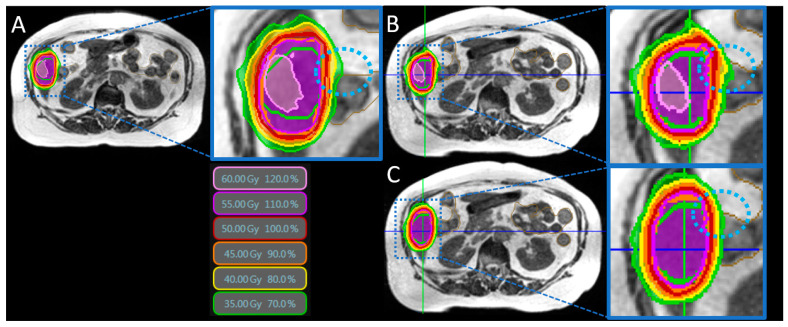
Case study. (A) Baseline plan (50 Gy in 10 fractions) with already slightly impaired PTV coverage (91%) due to proximity to the intestines (brown). (B) Daily online MRI-guidance revealed that the small bowel had moved slightly forward in ventral direction (dashed light-blue circle) leading to a predicted plan with excessive dose in the small bowel together with impaired PTV coverage (78 %). (C) Plan adaption led to the removal of the dose bulge (dashed light-blue circle) and resulted into superior sparing of the small bowel, while simultaneously improving PTV coverage (90%).
